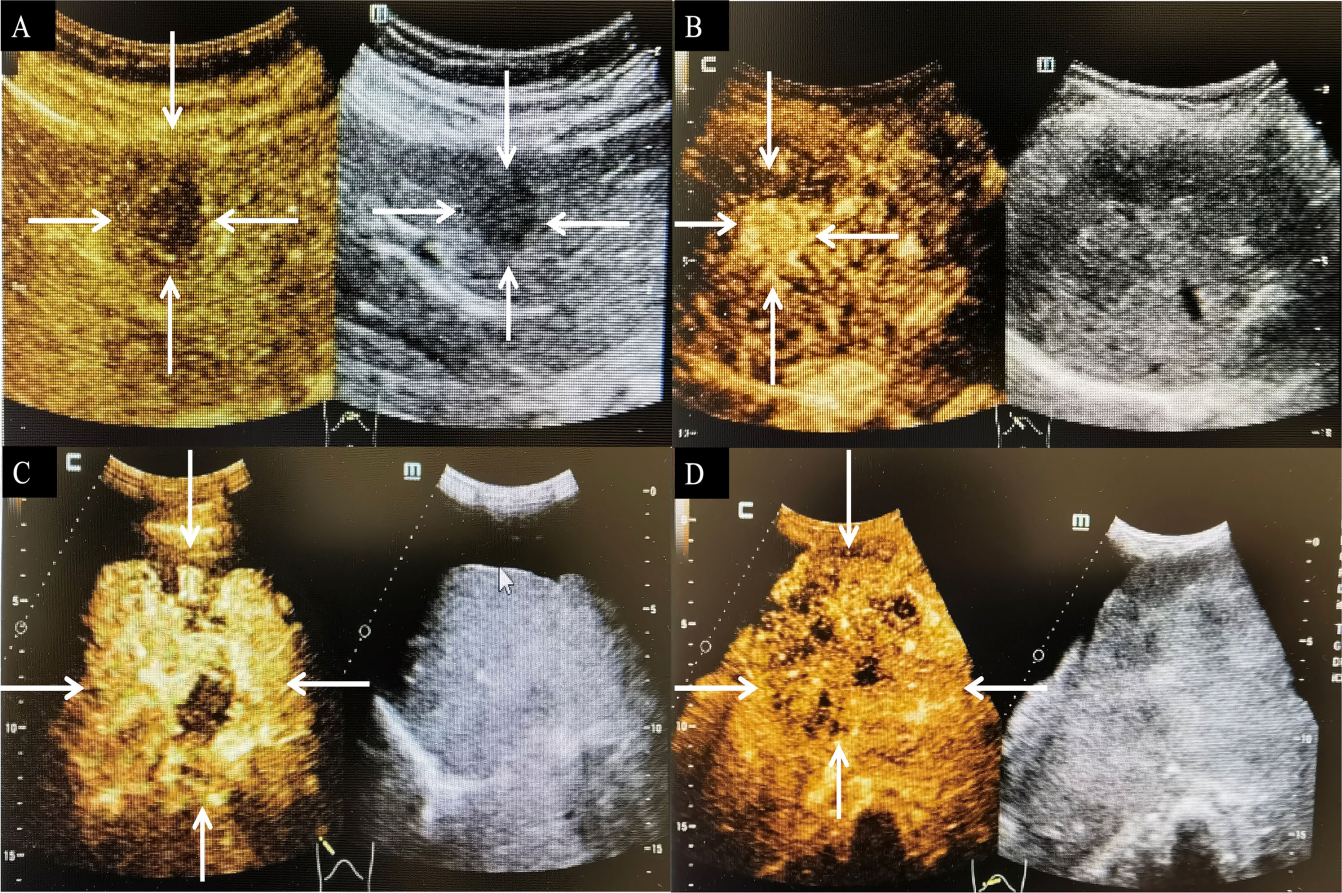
CEUS feature of HEHE. A 53-year-old female patient with hypoechoic nodule in the right lobe of the liver (arrows) (A) and a wholly uniformly enhanced nodule in the left lateral lobe (arrows) (B). A 33-year-old male patient with heterogeneous hyperenhancement at the arterial phase (arrows) (C) and hypoenhancement of the central part at portal and late phases (arrows) (D). CEUS, contrast-enhanced ultrasonography; HEHE, hepatic epithelioid hemangioendothelioma.
